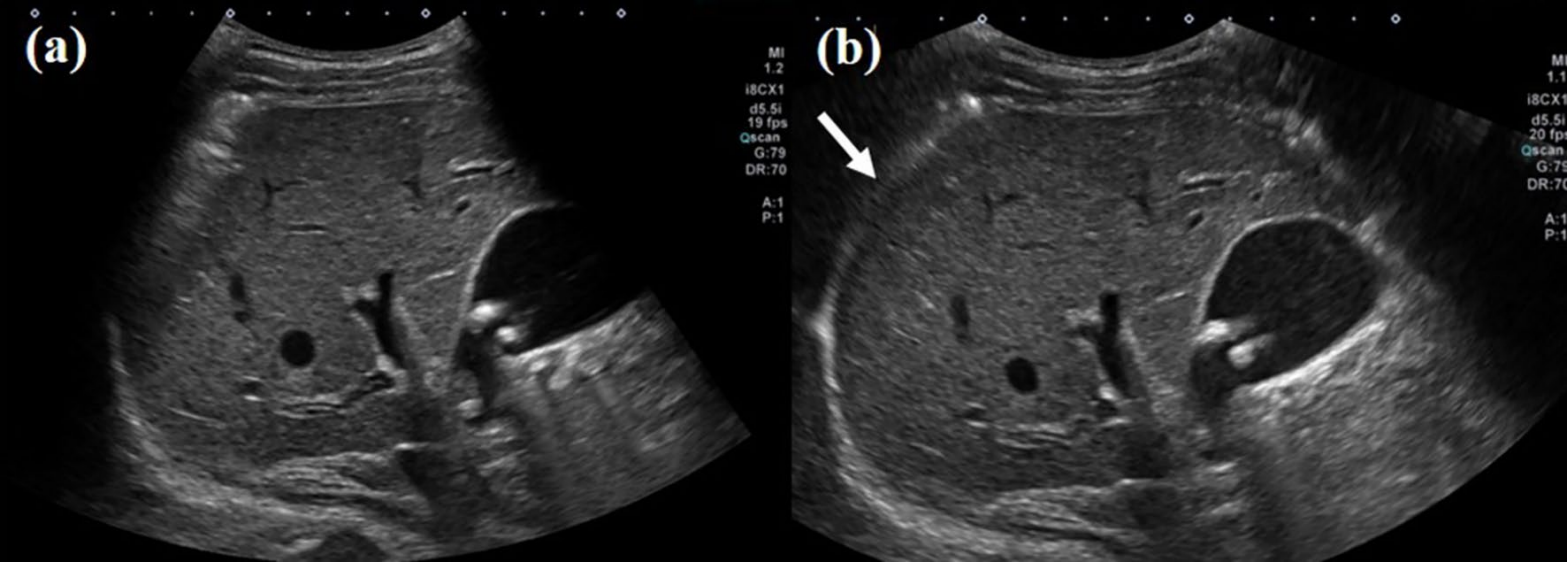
Ultrasound (US) images obtained from a 67-year-old male patient with chronic hepatitis B viral infection. a Intercostal scan of the liver right lobe using a routine view mode, displaying an incomplete view of the diaphragm with the subdiaphragmatic area obscured due to the limited field of view. b Intercostal scan of the liver right lobe using a wide view scan mode, providing a comprehensive view of the diaphragm edge, and clear visualization of the sub-diaphragmatic area (arrow)
